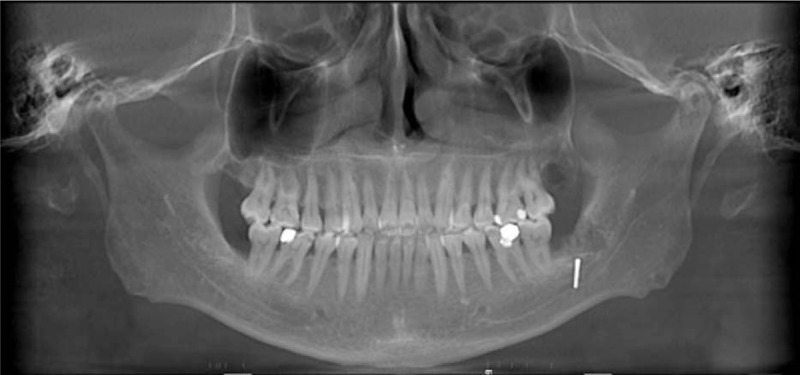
A metallic foreign body in the left lower jaw was presented in the orthopantomography.
